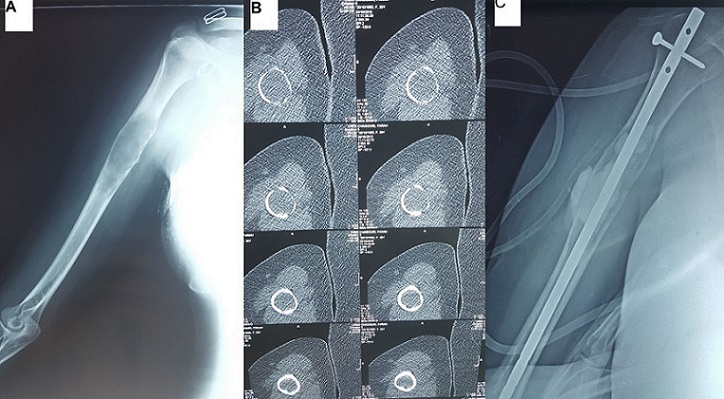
A) radiographie de l'humérus droit montrant une image lacunaire ostéolytique au niveau du tiers proximal; B) à scanner de l'humérus droit montant une image ostéolytique avec éffraction de la corticale; C) radiographie de controle de l'humérus droit après biopsie éxerese de la tumeur associée à une ostéosynth
